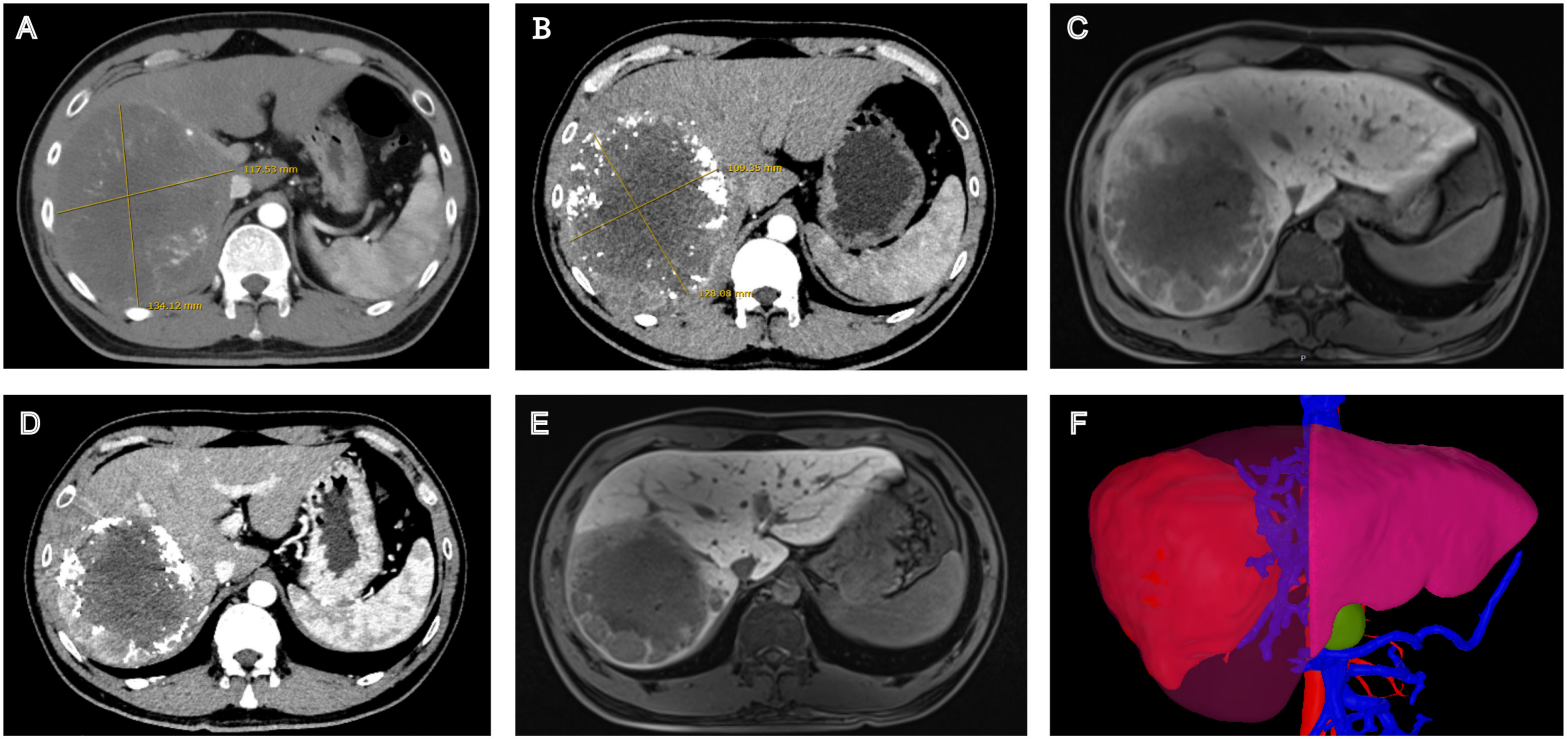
Radiological changes during the patient’s treatment course. (A) Contrast-enhanced CT at baseline. (B) Contrast-enhanced CT After c-TACE therapy. (C) Gd-EOB-DTPA-enhanced MR after c-TACE therapy. (D) Contrast-enhanced CT after sintilimab (3 cycles) and Tislelizumab (4 cycles). (E) Gd-EOB-DTPA-enhanced MR after sintilimab (3 cycles) and Tislelizumab (4 cycles). (F) Three-dimensional volumetric analysis based on contrast-enhanced CT before surgery.
